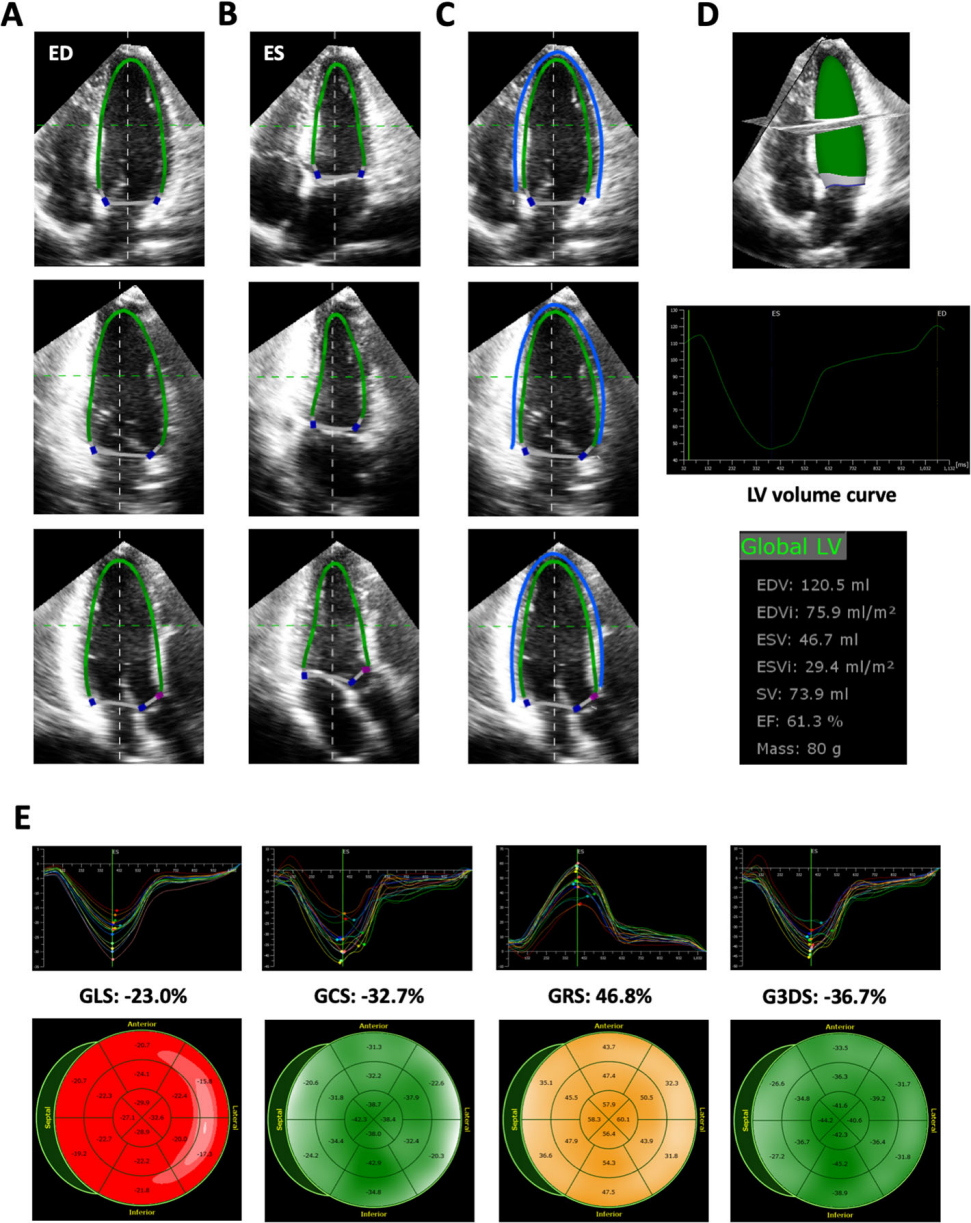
3D strain analysis using TomTec software in a healthy subject. Left ventricular endocardial border determination on the apical 4-, 2-, and long-axis views at end-diastole (a) and end-systole (b). Manual epicardial border determination resulting in LV mass analysis (c). Green lines indicate the endocardial border, and blue lines indicate the epicardial border. The software performed 3D speckle tracking analysis on the endocardial border, generating an LV volume curve, from which LV end-diastolic volume, end-systolic volume, and LV ejection fraction (LVEF) were calculated (d). The software also provided 3D regional and global longitudinal strain curves, a circumferential strain curve, a radial strain curve, and a 3D principal strain curve. Corresponding bull’s eye maps were also provided (e). ED(S)Vi, left ventricular end-diastolic (end-systolic) volume index; G3DS, global principal strain. Other abbreviations are the same as in Fig. 2
